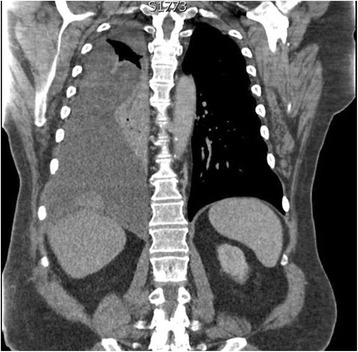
CT chest transverse view displaying right-sided pleural mass associated with significant unilateral pleural effusion
